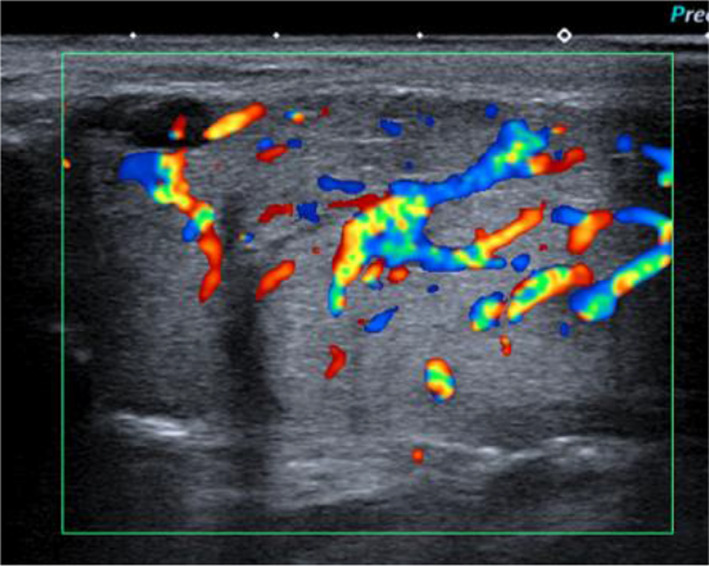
Acute viral right parotitis. Enlarged, hypervascularized parotid on ultrasonography
